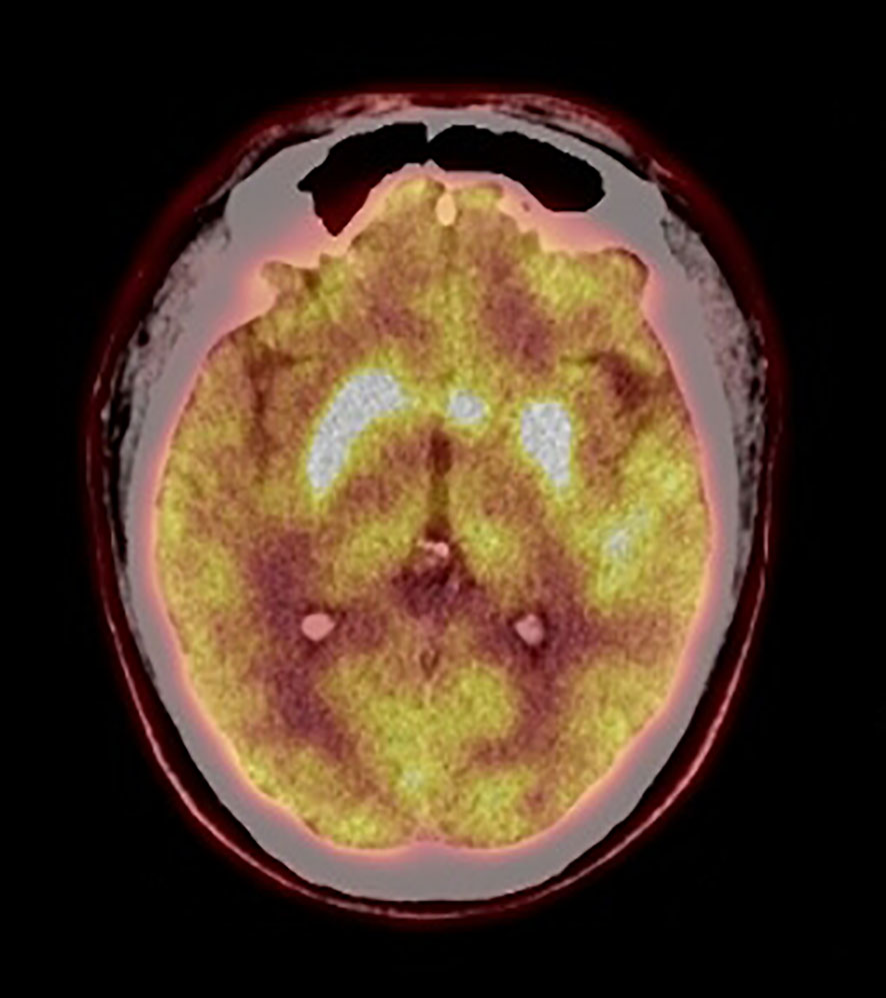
PET-CT imaging. PET-CT showed hypermetabolism level of bilateral caudate nucleus, putamen, and left temporal lobes.
